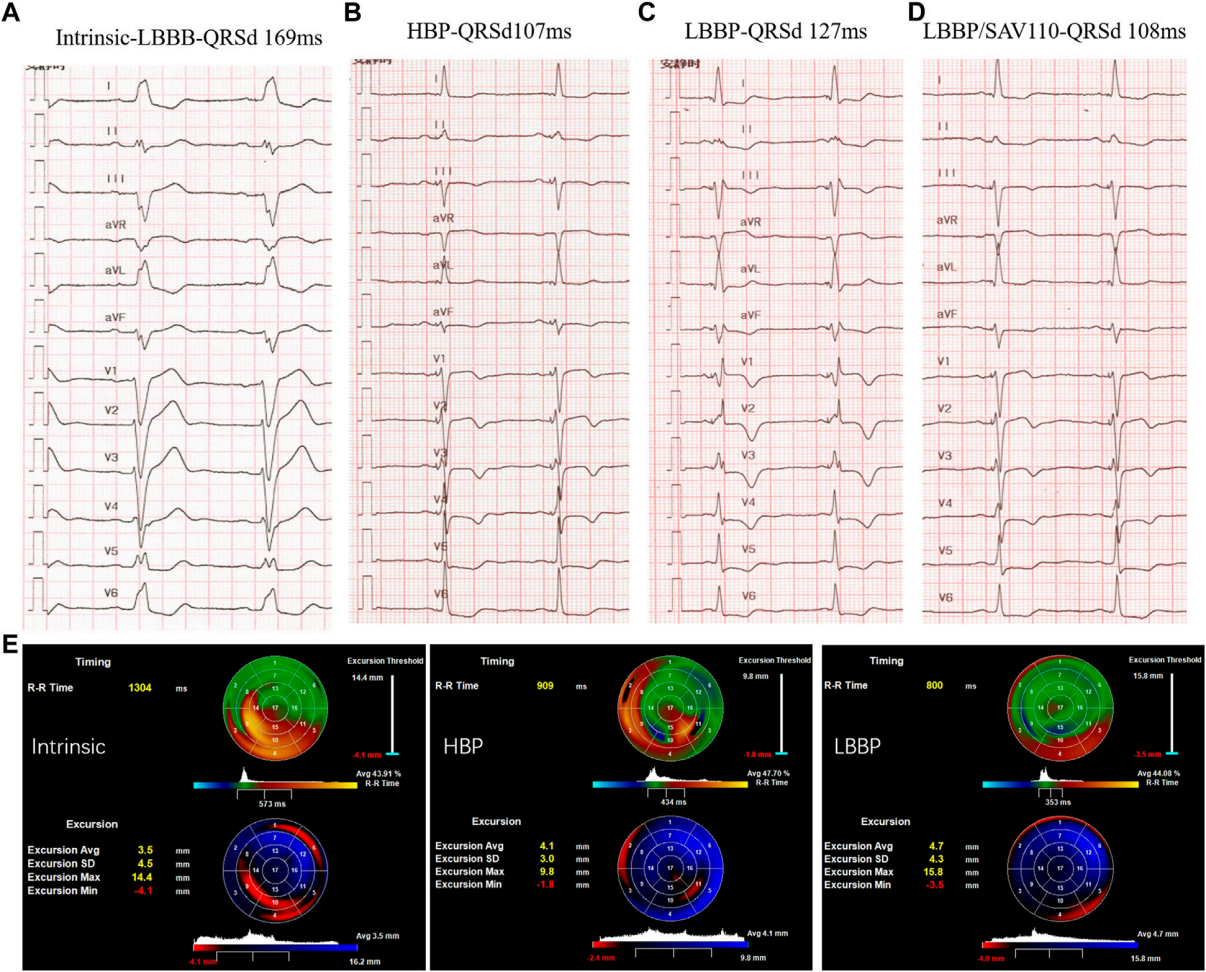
Comparison of LBBP and HBP in electrical and mechanical synchrony in a HF patient with LBBB: (A) Intrinsic rhythm with QRS duration (QRSd) of 169 ms; (B) Selective HBP at left bundle branch block (LBBB) correction threshold of 3 Vat 0.5 ms with QRSd equal to 107 ms. (C) LBBP at 0.5 V at 0.4 ms with QRSd of 127 ms and RV conduction delay pattern. (D) LBBP in DDD mode with SAV delay 110 ms with QRSd reduced to 108 ms. (E) 3-dimensional echocardiogram between selective His bundle pacing (HBP) and left bundle branch pacing (LBBP). Right: intrinsic rhythm; Middle: HBP in VVI mode; Left: LBBP in VVI mode. The upper “bull’s eye” depicts the timing of contraction green areas represent synchronous areas, blue areas contract early and red/yellow areas show late contraction. The lower “bull’s eye” shows the wall excursion with dark/red areas referring to reduced excursion and bright blue areas indicating the largest systolic radial excursion.
